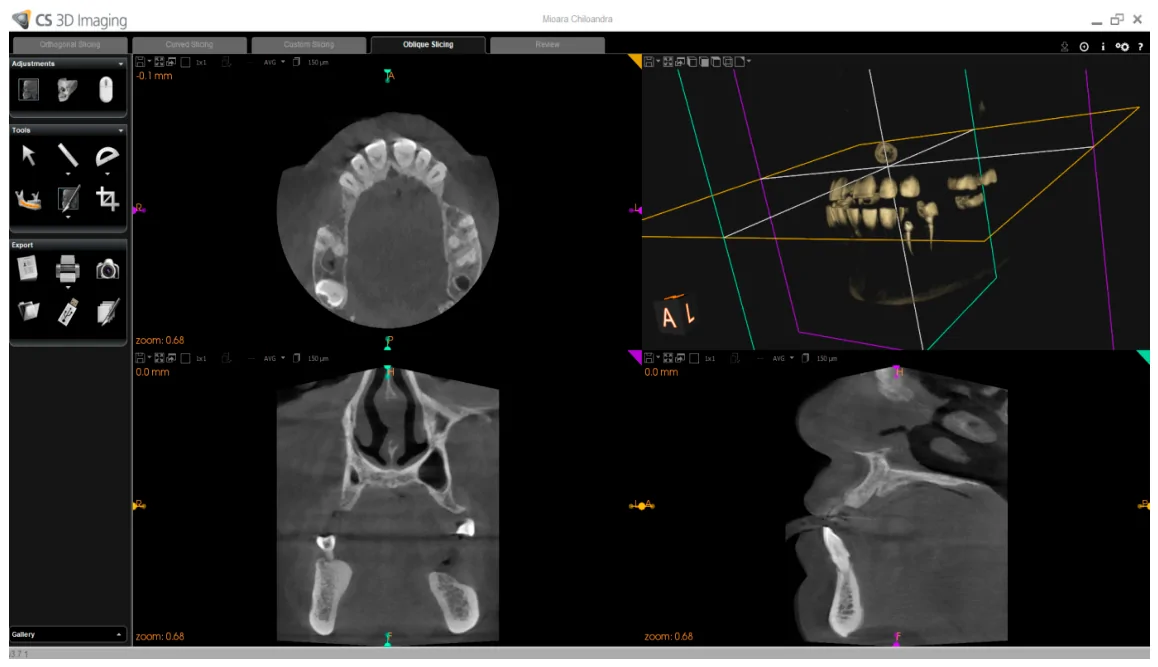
CBCT images of the patient under study.
